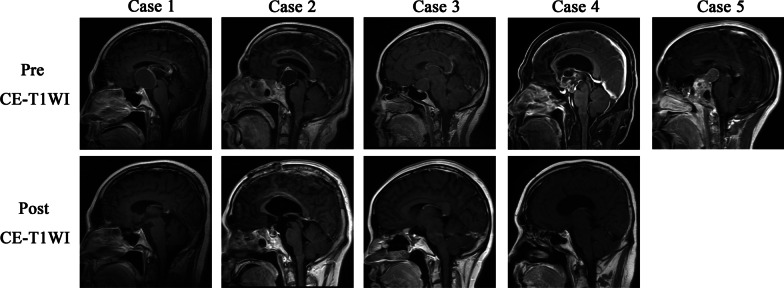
Pre- and postoperative magnetic resonance imaging of patients. The first line is the preoperative sagittal CE-T1WI MRI sequence. The second line is the sagittal CE-T1WI image obtained 3 months postoperatively, which is blank for patient 5 because the patient did not undergo surgery
